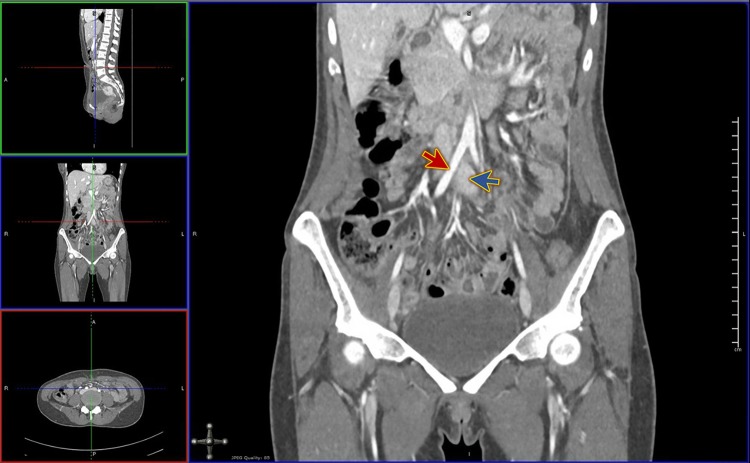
CT venogram coronal view shows compression of the left iliac vein (blue arrow) by the right iliac artery (red arrow).
